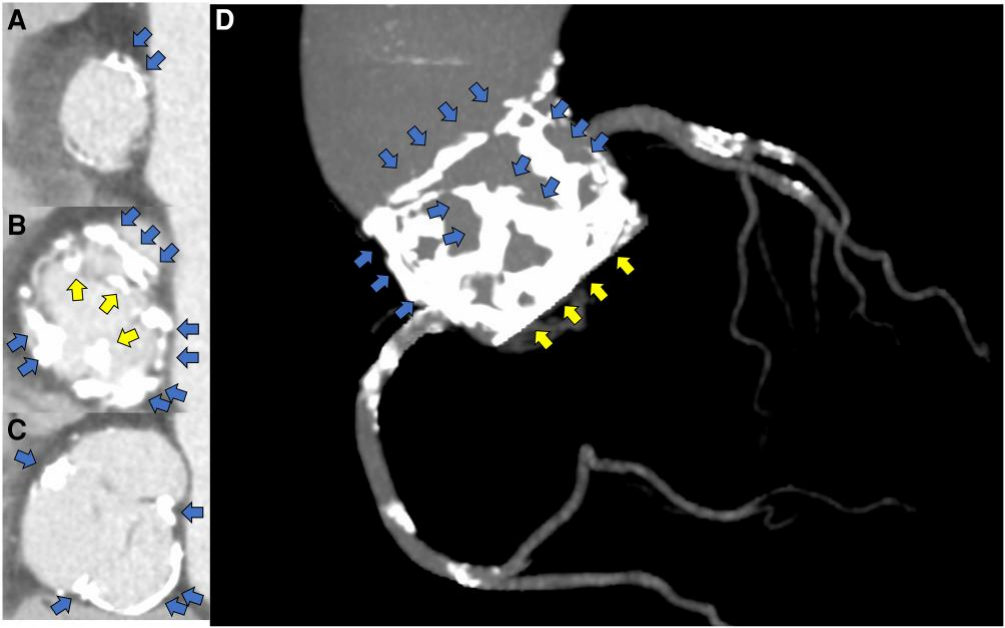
Pre-procedural computed tomography. (*A*) Annulus level, (*B*) 4 mm above the annulus, (*C*) sinus of Valsalva, and (*D*) 3D constructed aortic image. Blue arrows show the calcification of anastomosis. Yellow arrows show leaflet calcification. D, dimension.
